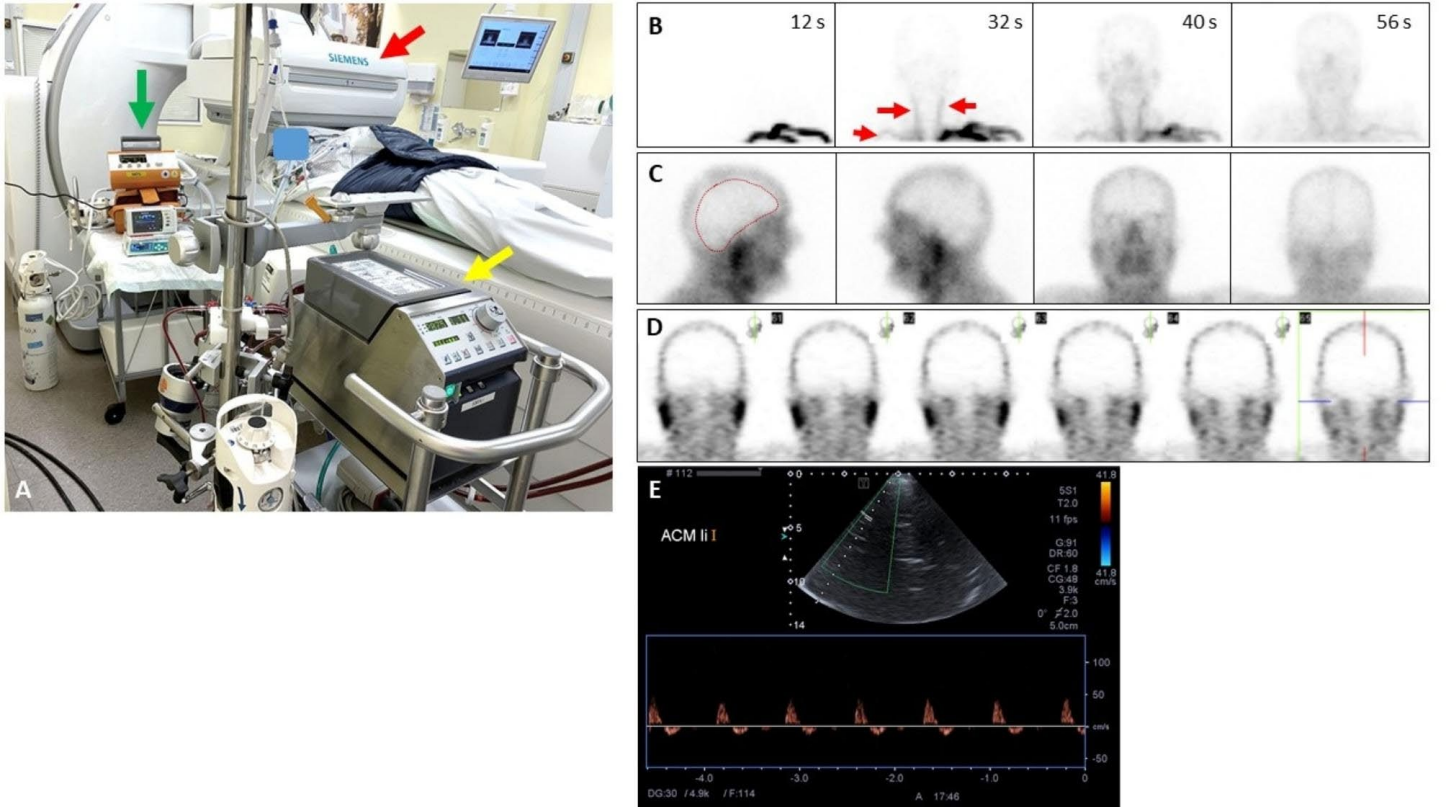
Imaging setup for perfusion scintigraphy with ^99m^Tc-HMPAO of the patient on vaECMO (A), with gamma camera (red arrow), portable respirator (green arrow) and ECMO pump/oxygenator (yellow arrow). The dynamic acquisition (B) shows inflow of the tracer from the left arm (12s after injection), appearing in the carotid and subclavian arteries 32s after tracer injection (red arrows) and in the extraaxial soft tissues of the head. Static planar images acquired after 30 min (C) do not show tracer uptake in the brain (dotted line), which can be confirmed by tomographic SPECT imaging (D, examplary coronal views). Transcranial Duplex-sonography (E). Circulation arrest in the left middle cerebral artery (MCA). Right MCA, distal carotid artery, bilateral V4 segments and basilar artery showed the same pattern
